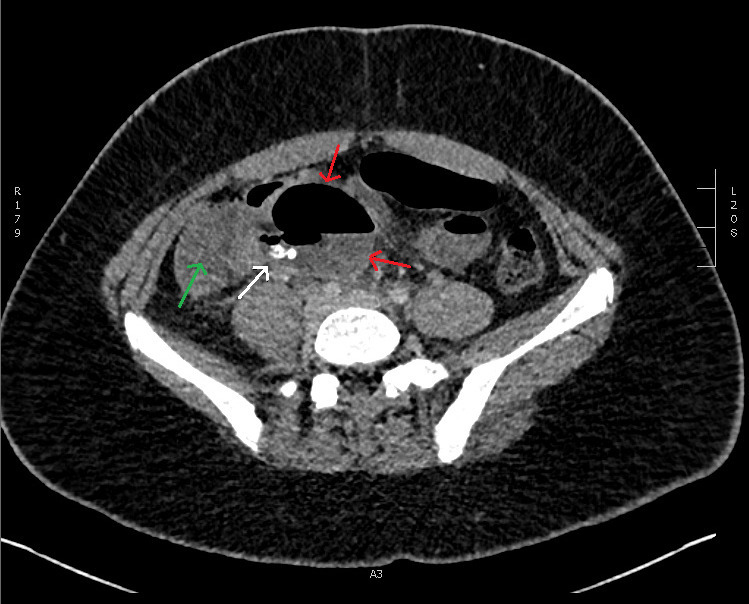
Preoperative CT (axial plane) demonstrating appendix rupture with a gas
